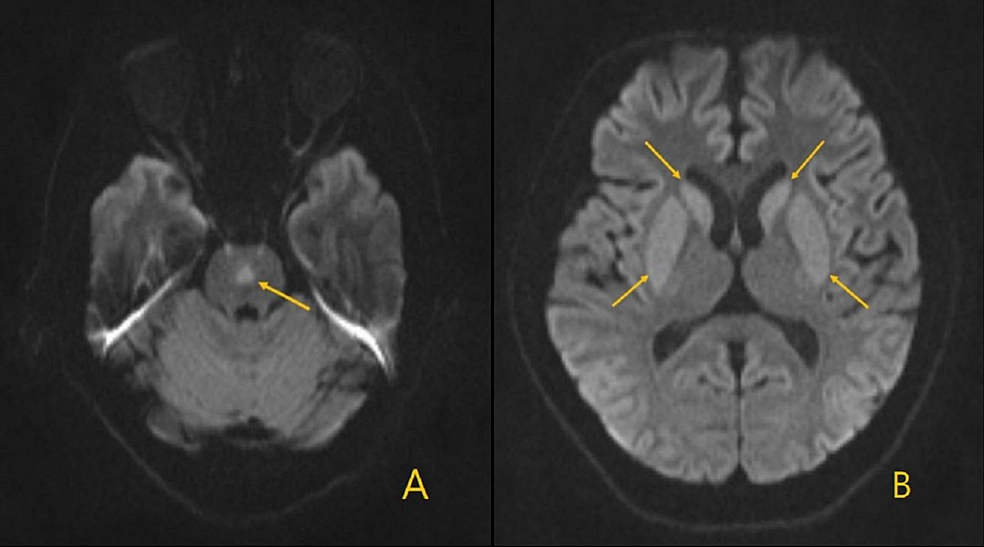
Brain MRI (DWI) on day 12 of hospital admission. The axial view of the DWI shows: (A) new high intensity lesion at the central pons, consistent with CPM; and (B) the previous lesions at the bilateral caudate nucleus and putamen did not subside and became more conspicuous. DWI: diffusion-weighted imaging; CPM: central pontine myelinolysis
